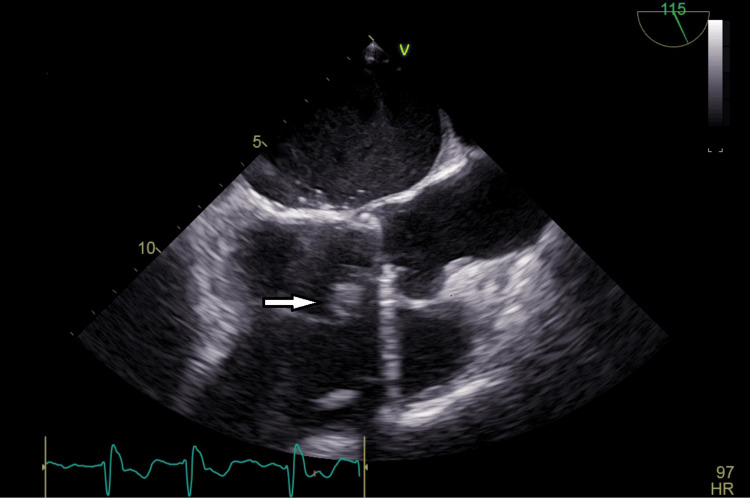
Echocardiography demonstrating a vegetation (white arrow) over the aortic valve
